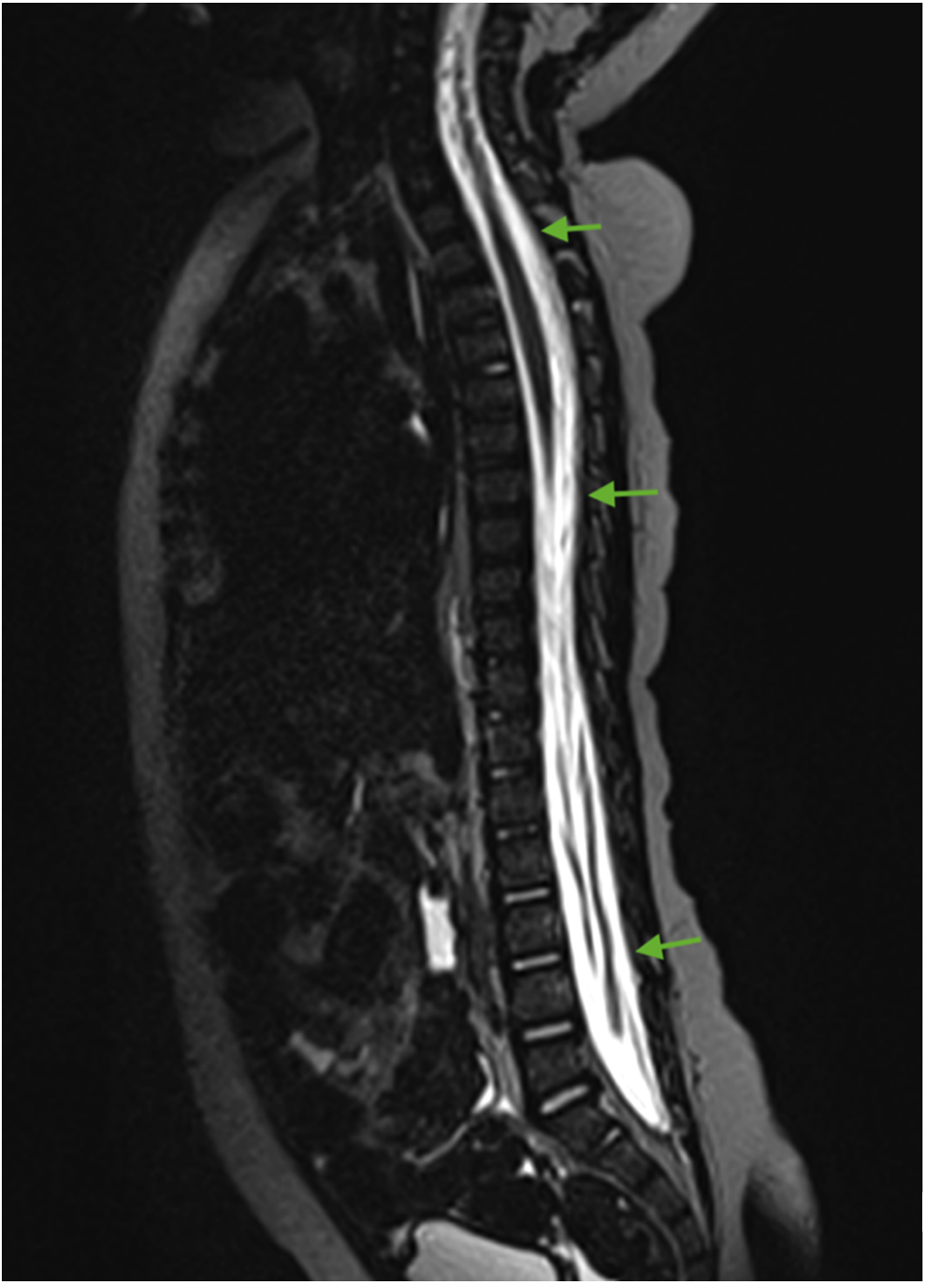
Spinal cord MRI (sagittal T2 + C). Thickening of the dura mater and intense contrast accumulation along the whole spine (green arrows).
